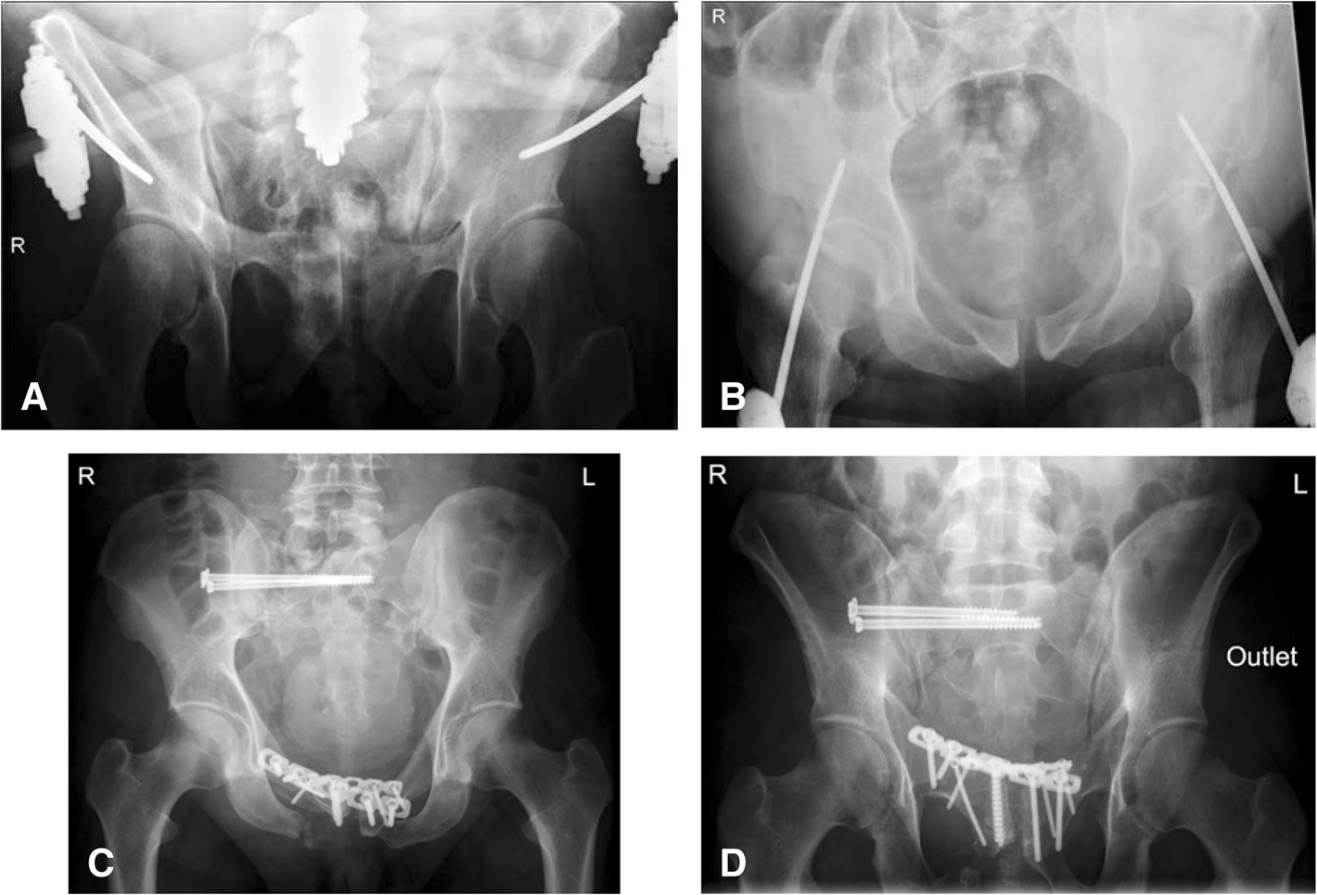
Postoperative plain pelvic radiographs. Temporary fixation of the anterior pelvis with supraacetabular external fixator (a, b) shows good reduction of the pubic symphysis. After definitive osteosynthesis iliosacral screws and anterior plating stabilize the pelvic ring (c, d)
